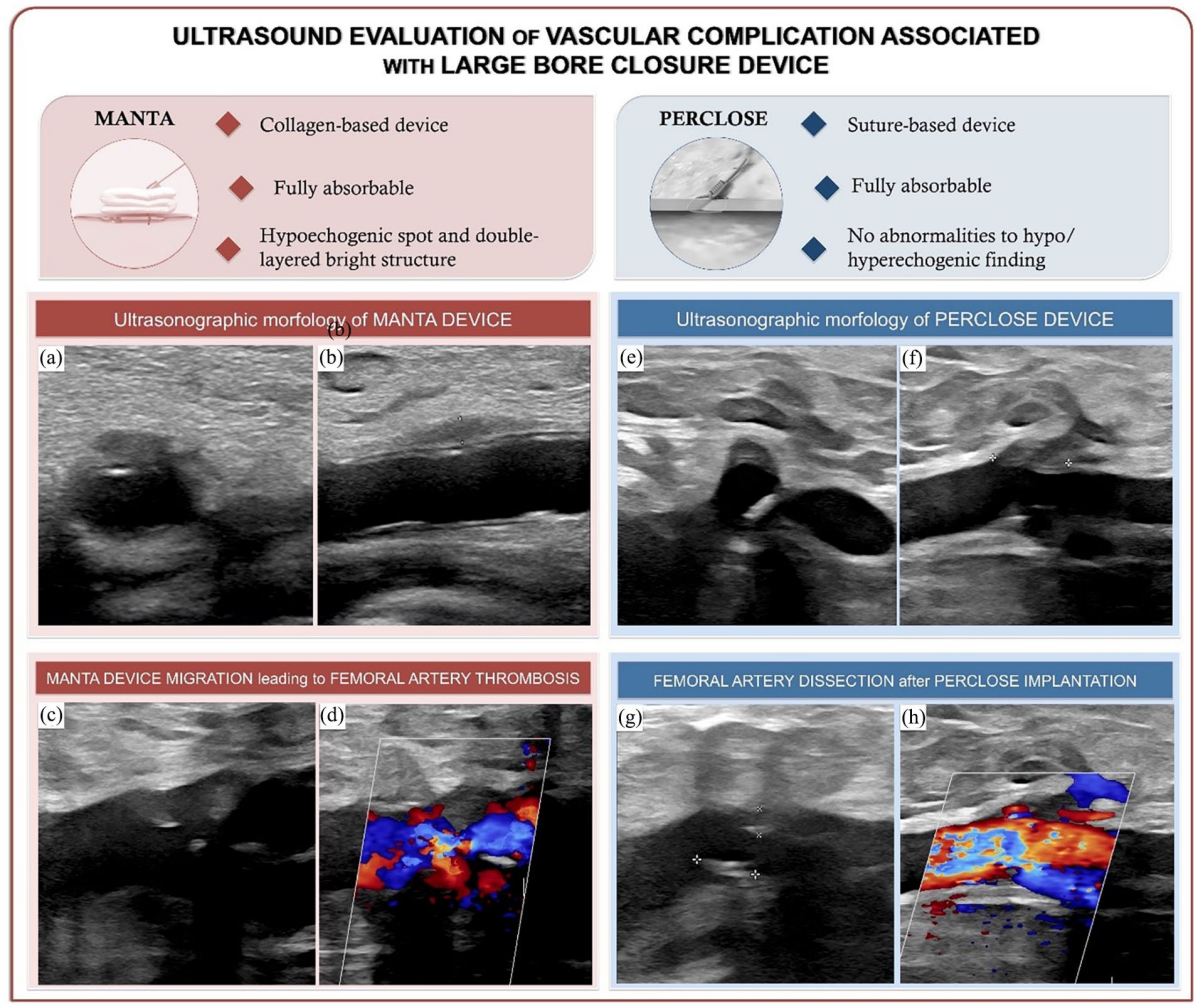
Ultrasound evaluation of large bore closure devices’ vascular complications. Common femoral artery (CFA)’s transverse (a) and longitudinal (b) ultrasound (B-mode) images showing MANTA’s normal ultrasound characteristics (a double-layered bright structure in the lumen formed by the poly-lactate toggle, and a perivascular hypoechogenic area corresponding to the collagen placement). Longitudinal ultrasound image (B-mode) (c) showing MANTA displacement leading to partial obstructive CFA thrombosis and hemodynamic stenosis on color Doppler ultrasound (d). Transverse (e) and longitudinal (f) ultrasound (B-mode) images showing no significant abnormalities of the CFA vessel wall in correspondence with Perclose, except for a hypoechoic shadow in the surrounding tissue, compatible with the introducer track. In presence of vessel diffuse atherosclerosis and vascular calcifications, the use of Perclose has resulted in a CFA dissection, with a visible intimal flap (highlighted in the image by cursors) (g) and acceleration of blood flow at this level on the color Doppler ultrasound image (h).
